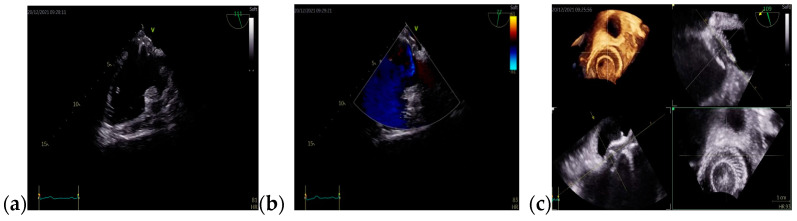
TOE: (a) Bicaval view: the Amplatzer occluder device (b) Mid-esophageal short-axis view: Amplatzer occluder device with no residual shunt (c) 3D TOE: Amplatzer occluder device; Abbreviations: TOE—transesophageal echocardiography.
